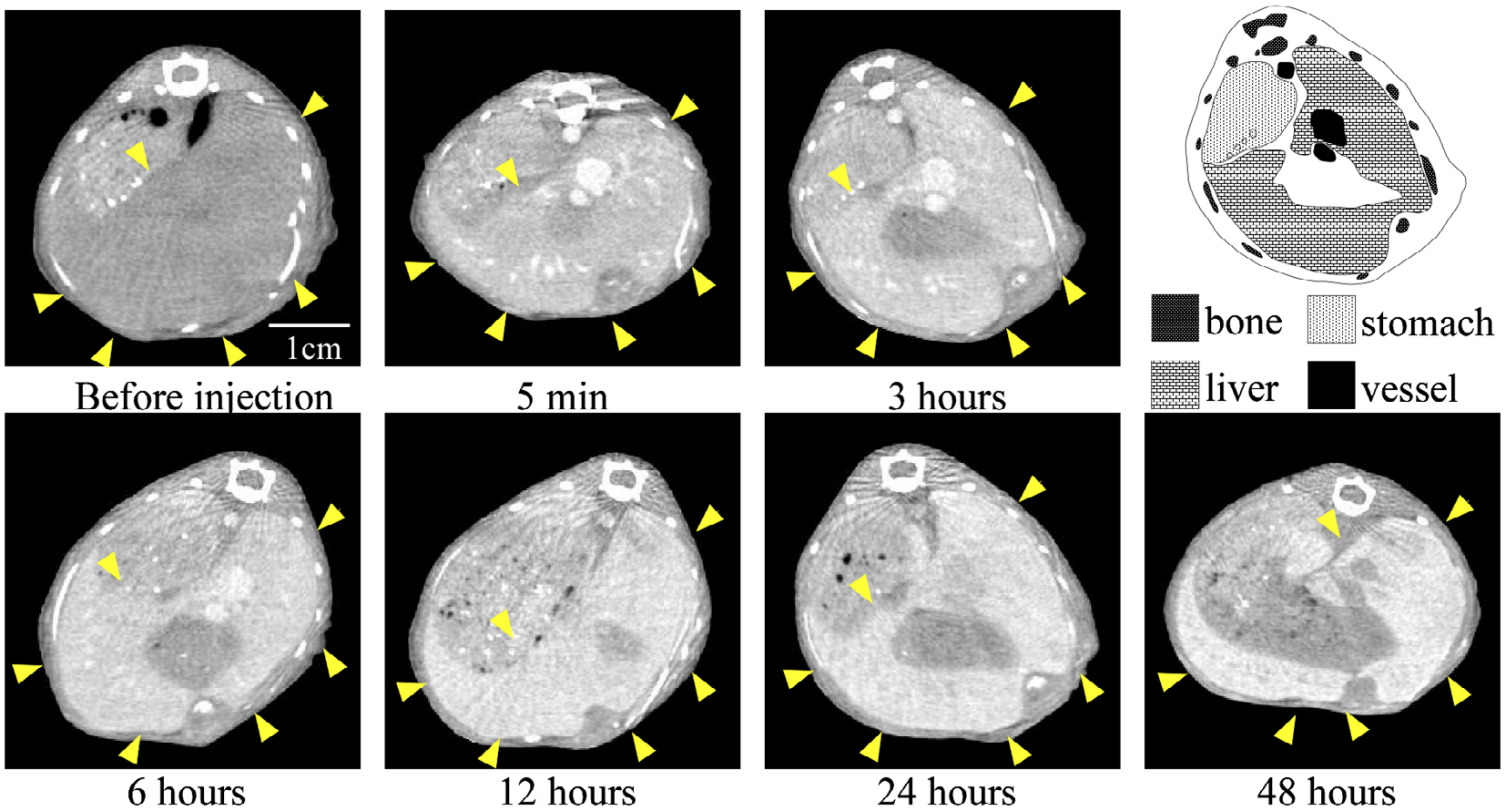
Change in CT value of a liver slice before and after injection of 30-nm Au-PEG. Yellow arrowheads indicate the region of the liver.
